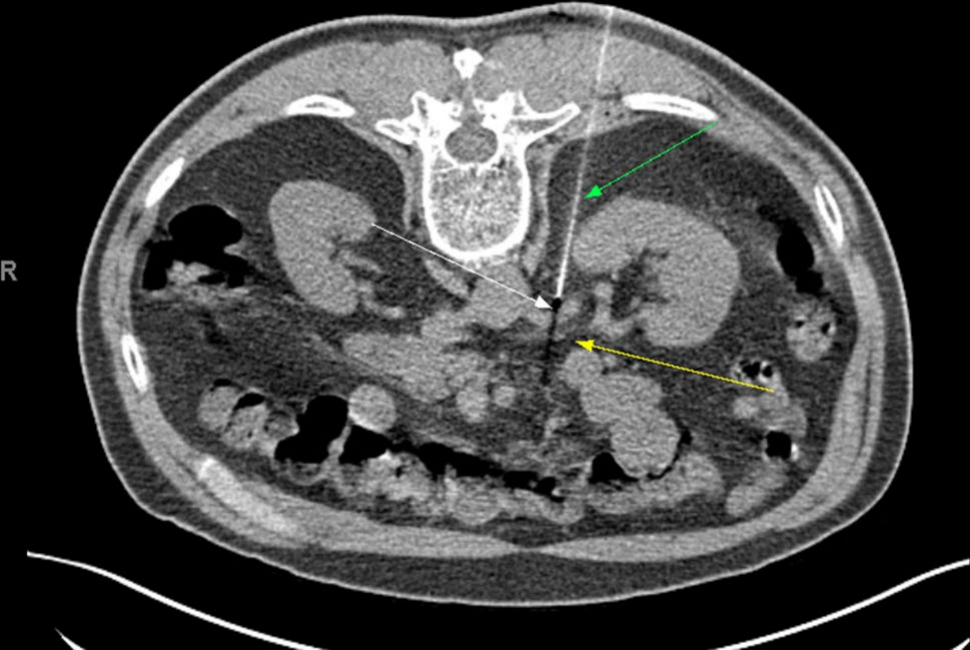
Renal hilar block under CT guidance. Green arrow—needle, white arrow—renal artery, yellow arrow—renal vein
